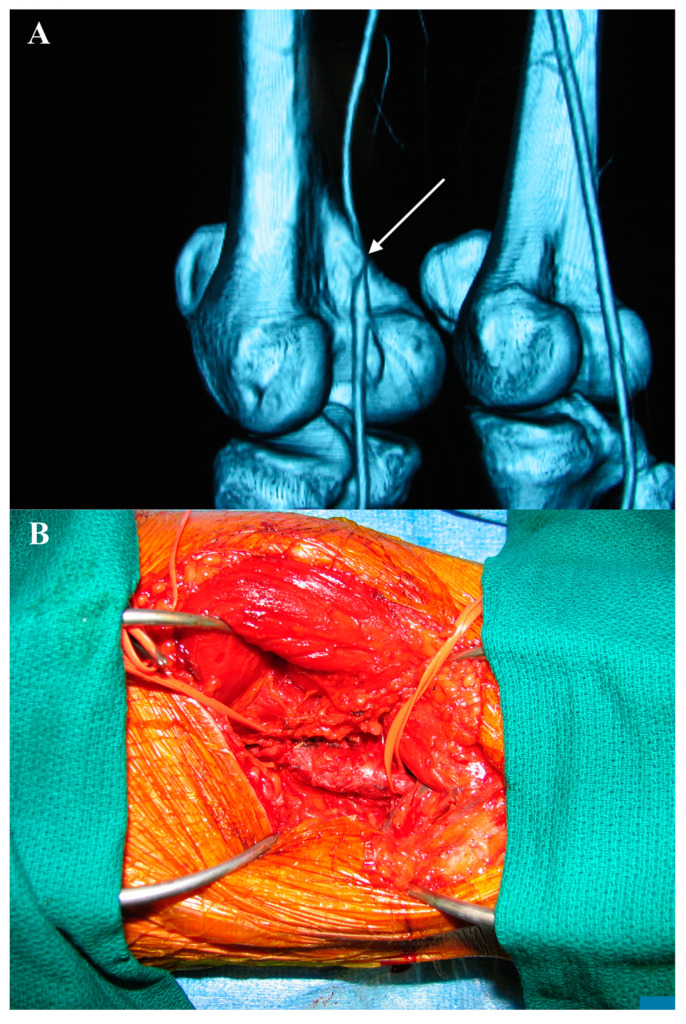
(A) CTA shows an entrapment of the left PA by a structure related to the arterial wall (white arrow). (B) The left PA is exposed by S-shaped skin incision in the popliteal fossa. An adventitial cyst is visible between the elastic loops.
